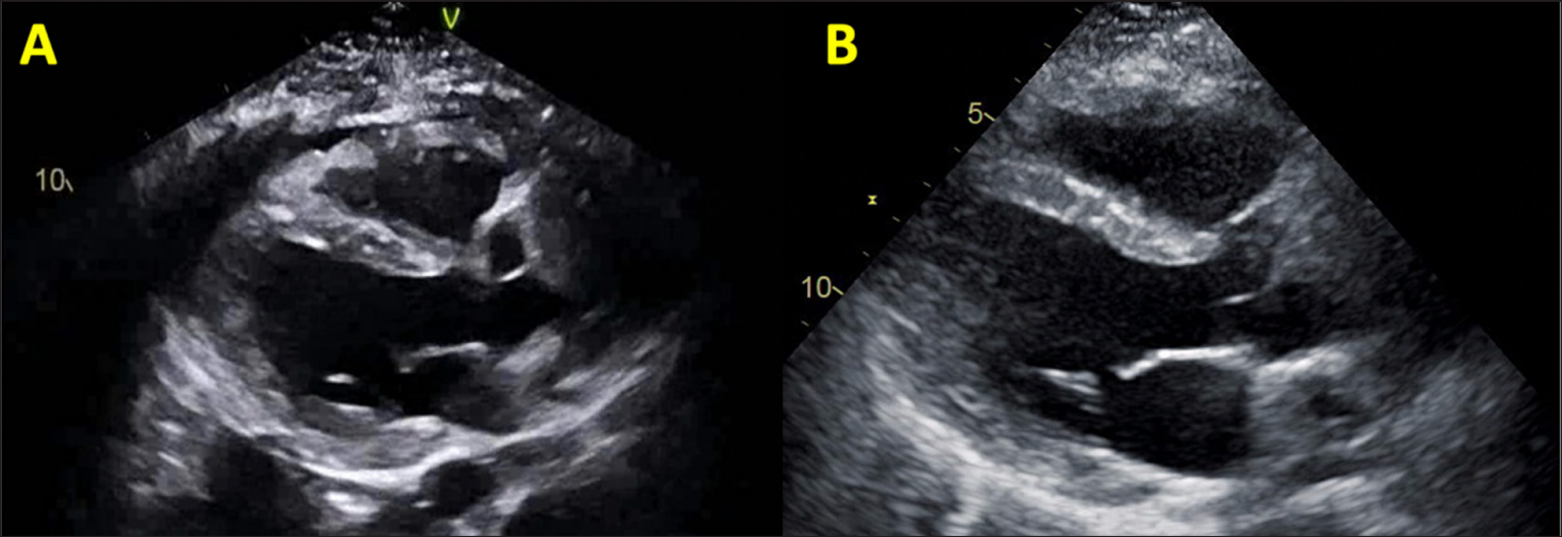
(A) Pretreatment echocardiogram (echo) with pericardial effusion, myocardial edema, and depressed left ventricular (LV) and right ventricular (RV) function. (B) Post-treatment echo with LV and RV recovery.
